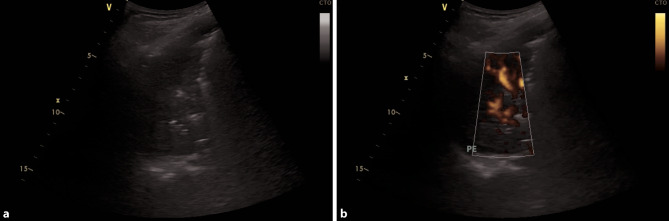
Bacterial superinfection of the left lower lung lobe in COVID-19. a Large consolidation with air bronchograms in a bacterial superinfection in COVID-19. b Same consolidation with power Doppler to display vascularization, a small pleural effusion is seen (*PE*)
